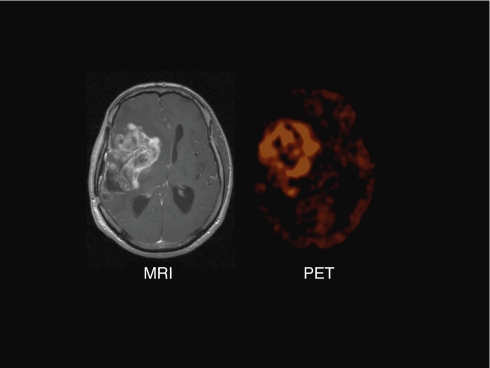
Brain MRI showing a large glioblastoma tumour with areas of necrosis within the tumour and significant brain oedema. On the right, a corresponding FDG-Glucose PET from the same patient shows much higher glucose uptake within the tumour, compared with the surrounding brain tissue.
